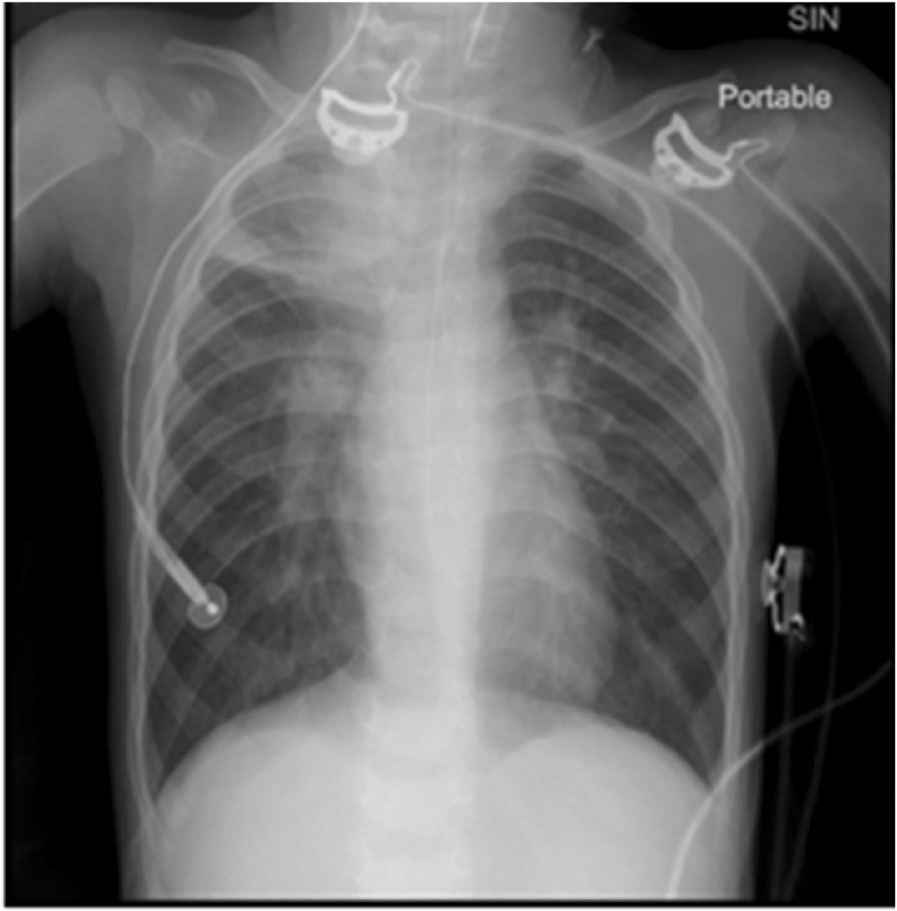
Chest radiograph (anteroposterior view) of the patient on the first day of hospitalization, showing upper right-side pneumonia
